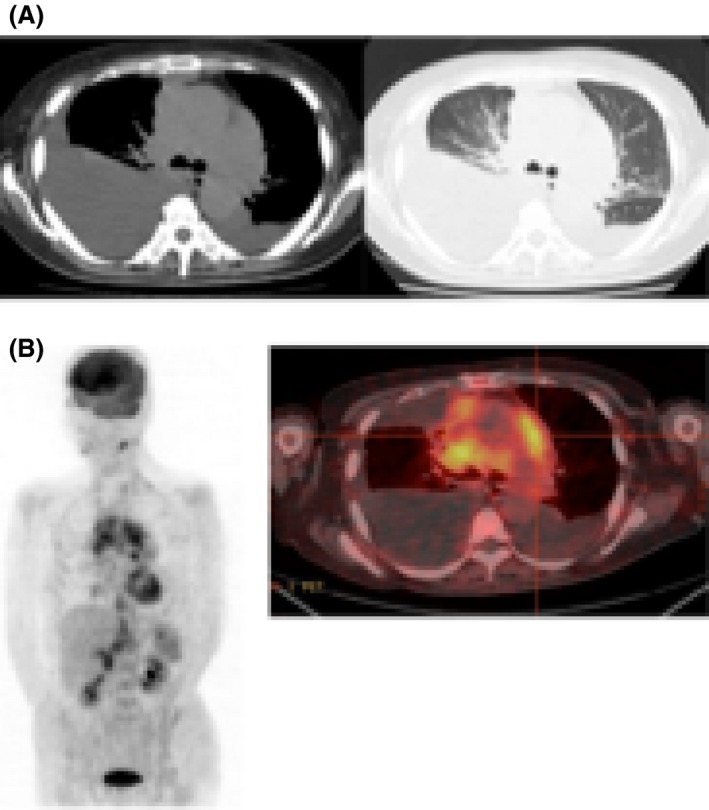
Imaging findings. (A) Computed tomography images at initial consultation. (B) FDG positron‐emission tomography images obtained before R‐CHOP. The image shows FDG accumulation in the cervical, mediastinal, hilar, and abdominal lymph nodes. FDG, 18F‐Fluorodeoxyglucose; R‐CHOP, Rituximab‐CHOP.
